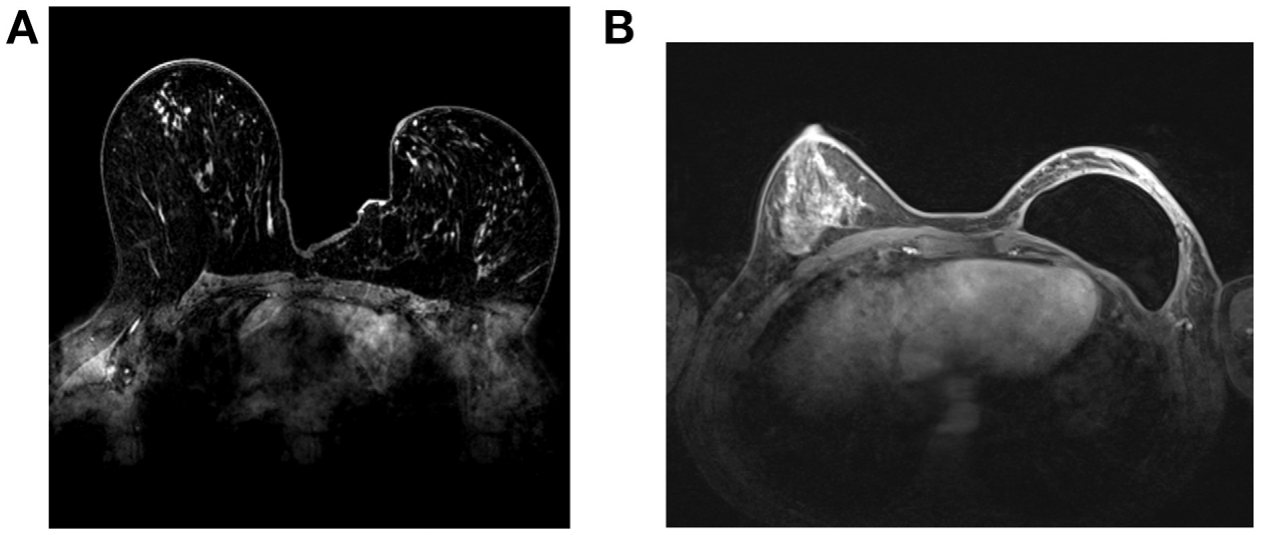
Breast MRI images at 1.5T. A Conventional breast MRI image (without contrast agent) (A). A breast prosthesis can be seen in the right breast (B).
